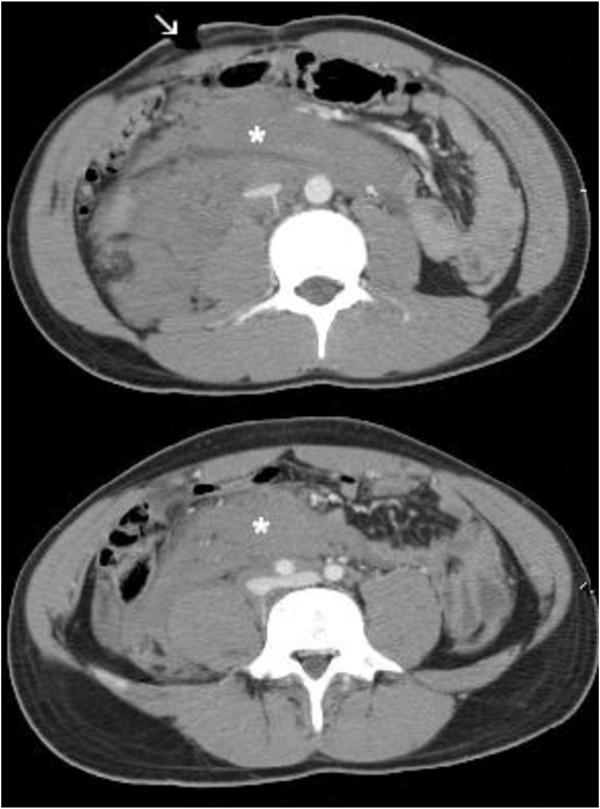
CT scan on admission. CT scan on admission showed a large retroperitoneal hematoma (*). Entrance site of penetrating wound is visible at right lower quadrant (arrow).
